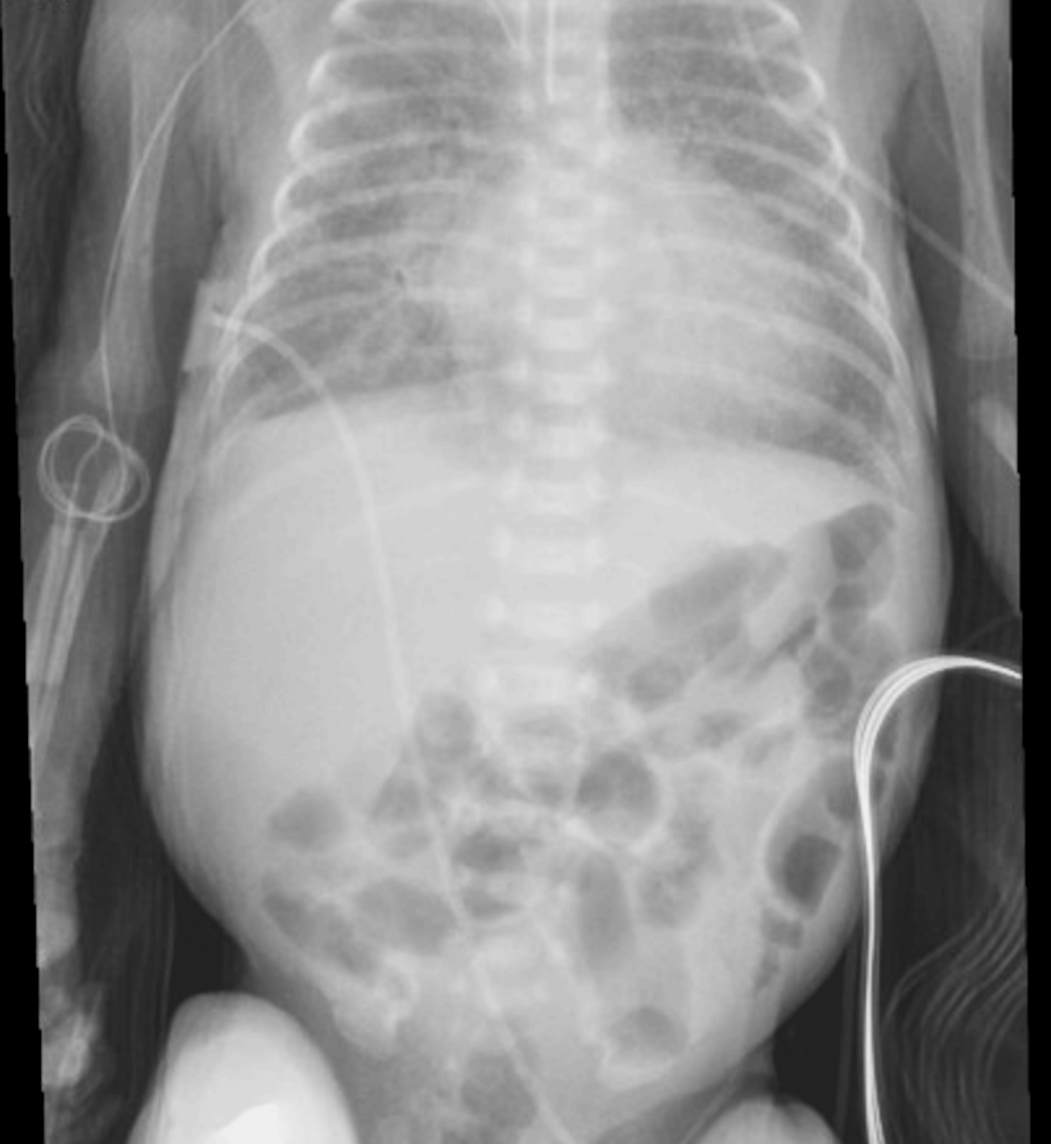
KUB radiograph showing improvement in gaseous distension of the stomach and small intestine following gastric tube decompression KUB: kidney, ureter, and bladder
